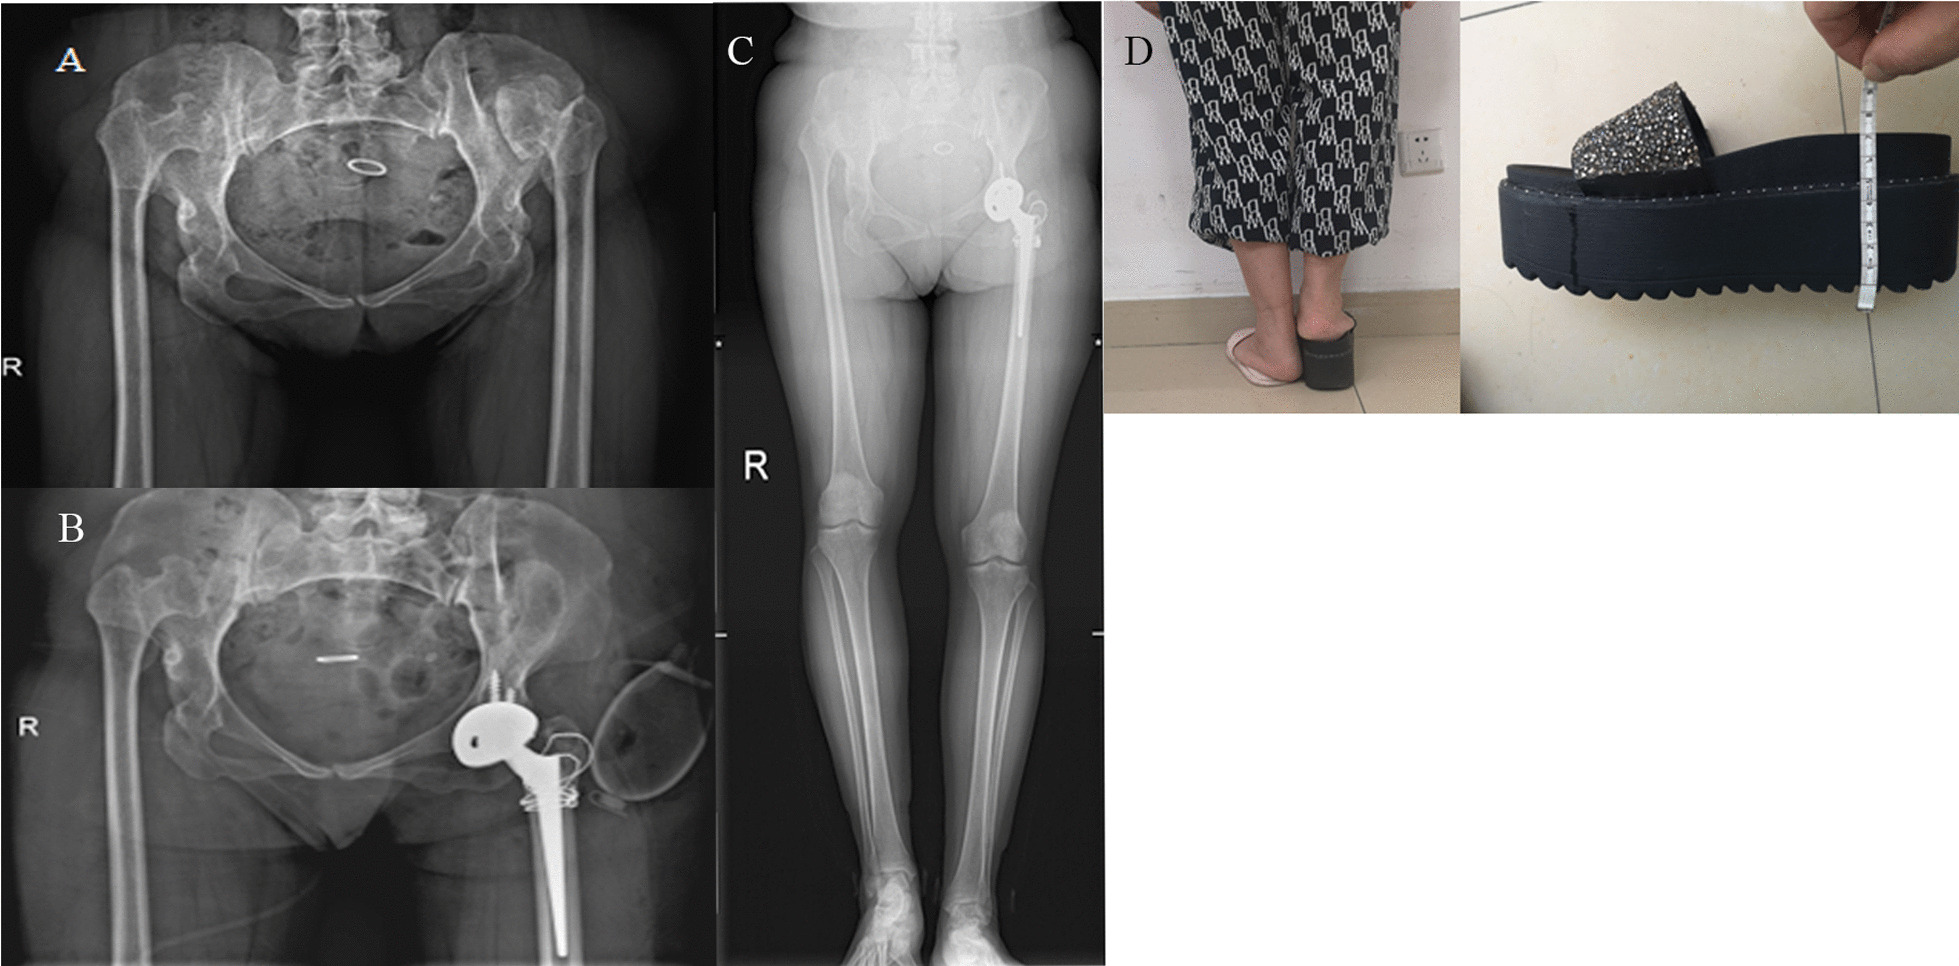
Stage-wise details of a 49-year-old female patient with bilateral Crowe type IV DDH who underwent THA surgery. A Preoperative X-ray exams of bilateral hip joints showed that both femoral heads were deformed and detached from the outside of the true mortar, which was shallow and small; B and C In the first stage, the left THA was performed first. The postoperative X-ray showed that the left acetabular prosthesis was in the true acetabulum, and the greater trochanter bone block was well fixed with steel wire; D Three months after the first surgery, the right limb was significantly shorter than the left, about 7.5 cm
